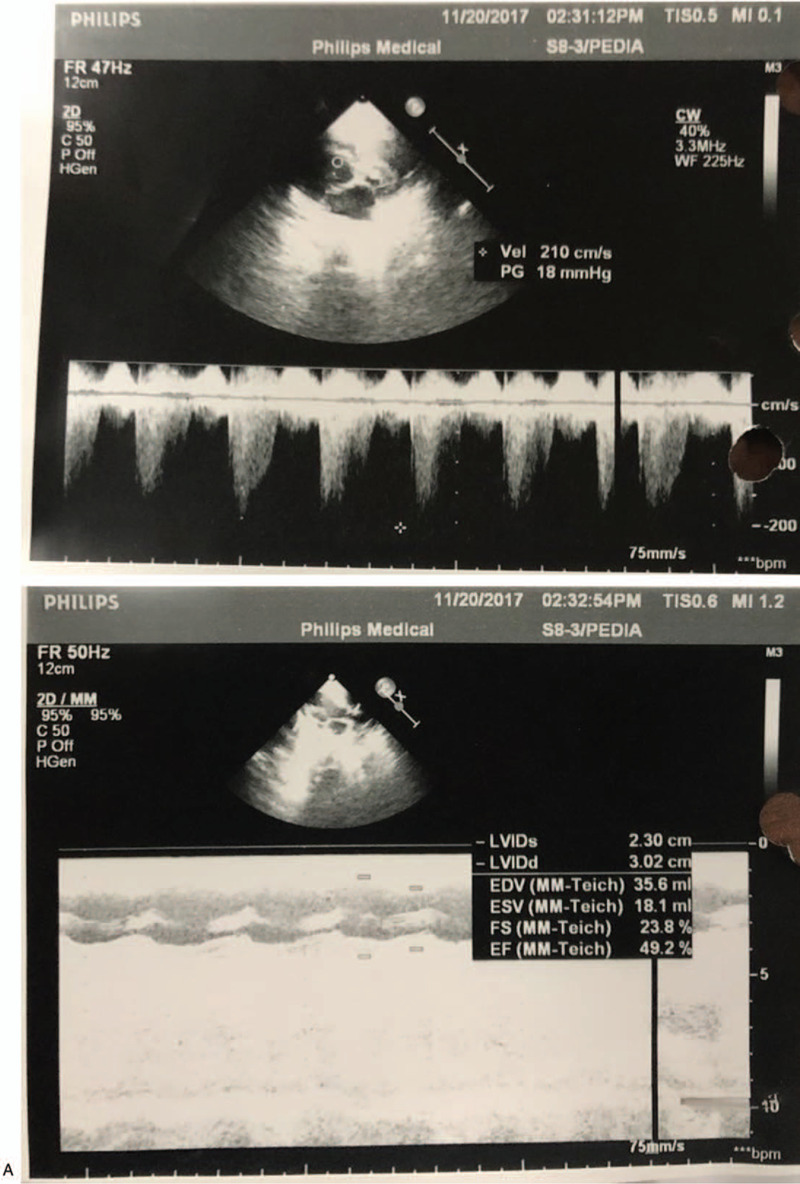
Echocardiography of the patient showing hypertrophic cardiomyopathy with mitral regurgitation, tricuspid regurgitation, and pulmonary hypertension.
